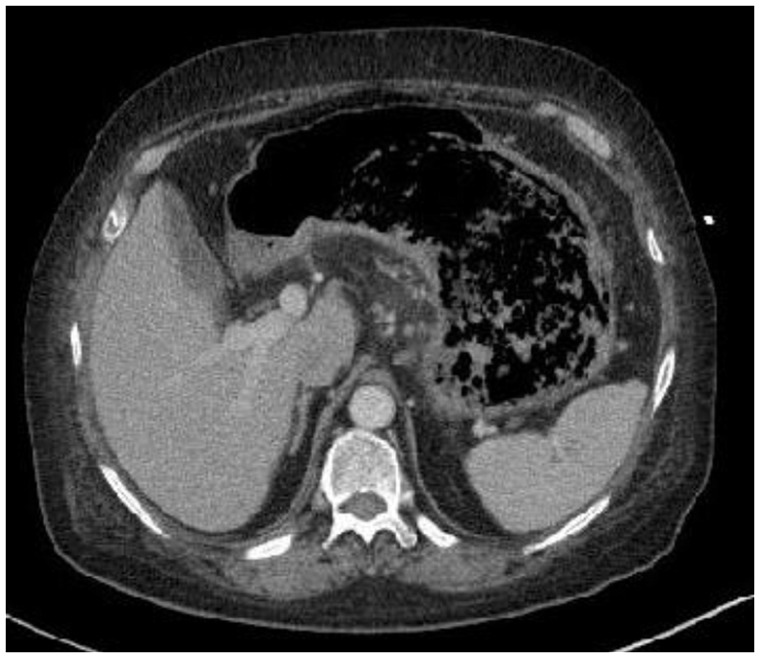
Post-contrast axial CT of the abdomen at the level of the gastric body reveals a large, loculated mass with a large amount of gas bubbles occupying most of the gastric body and fundus
